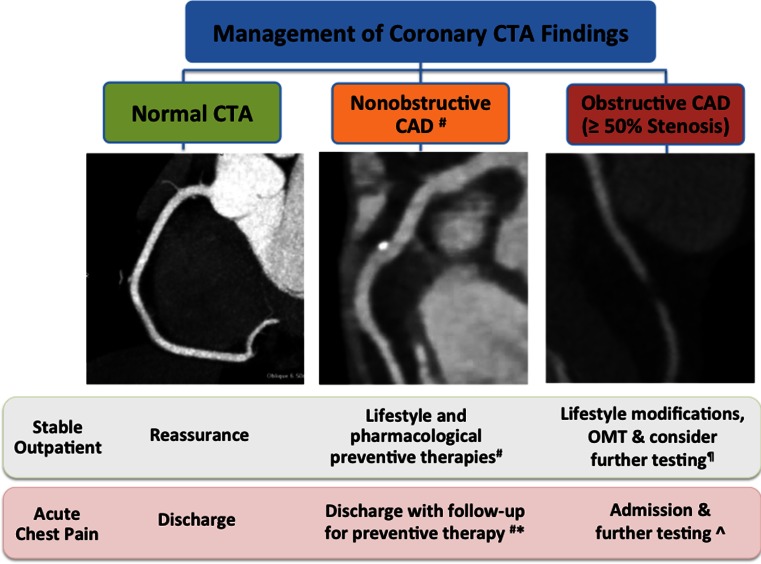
Management of coronary CTA findings. Patients with a normal coronary CTA have a very low (<1 %/year) rate of MACE and can be reassured while ED patients may be safely discharged. In patients with nonobstructive CAD, follow-up lifestyle modification and preventive therapy is recommended. *Number sign* revise risk upwards if diffuse nonobstructive CAD (e.g., segment involvement score > 4) and/or high-risk plaque features present (see Fig. 3). *For acute chest pain, consider repeat biomarkers prior to discharge if extensive nonobstructive CAD present. ^¶^In obstructive CAD with symptoms concerning for stable ischemic heart disease, first-line optimal medical therapy is recommended with consideration for further testing according to appropriate use criteria for testing after CTA (see Table 3) [65••]. ^In patients with acute chest pain and obstructive CAD, follow guidelines for the diagnosis and management of unstable angina/non-ST-segment elevation myocardial infarction to guide an early invasive versus conservative strategy [66]. *CAD*, coronary artery disease; *CTA*, computed tomographic angiography; *OMT*, optimal medical therapy
